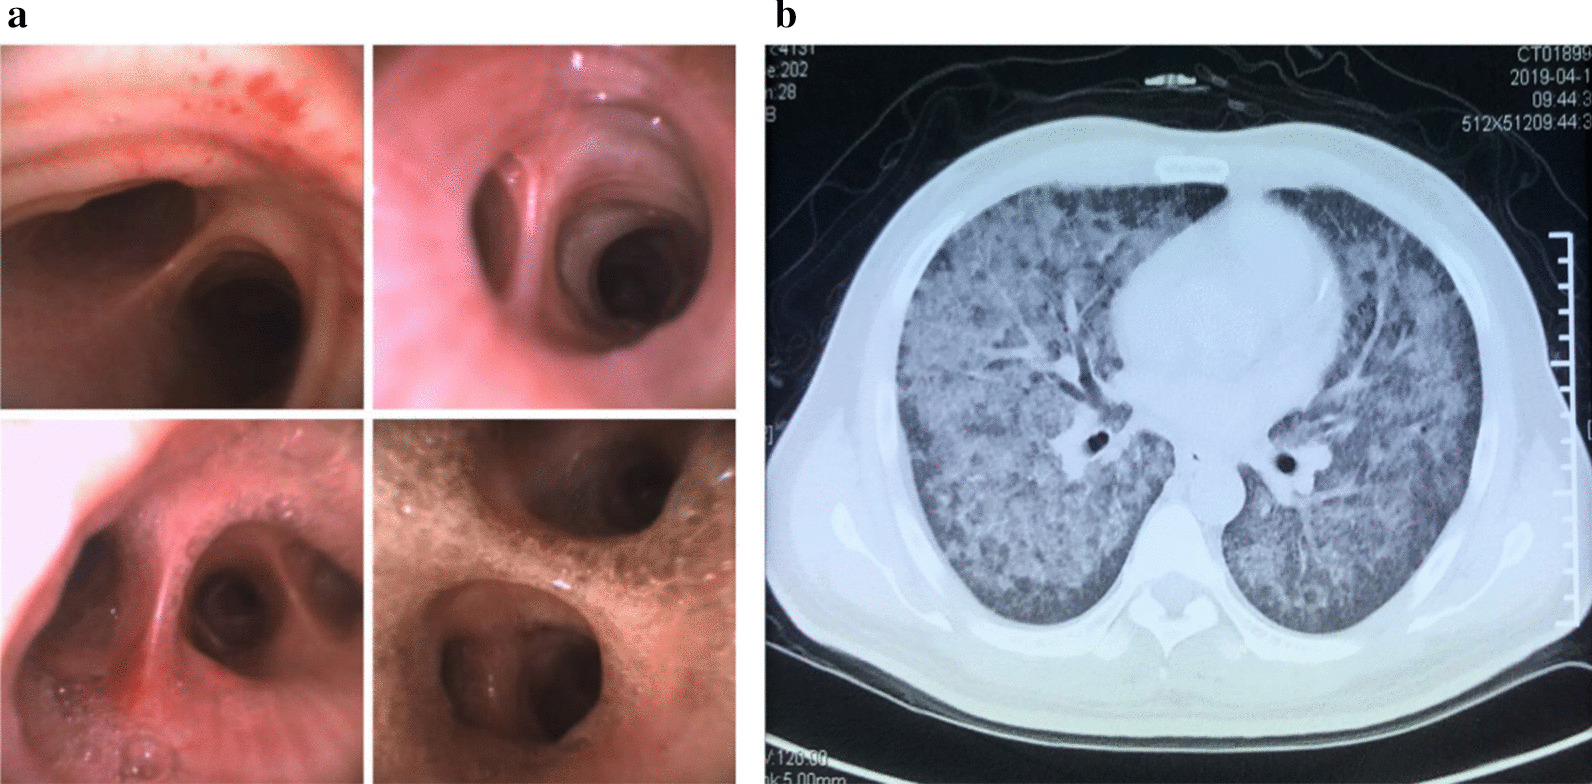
Bronchoscopy and chest computed tomography image. a. Images taken during bronchoscopy showing miliary nodules. b. Chest computed tomography image showing ground-glass opacifications in both lungs
